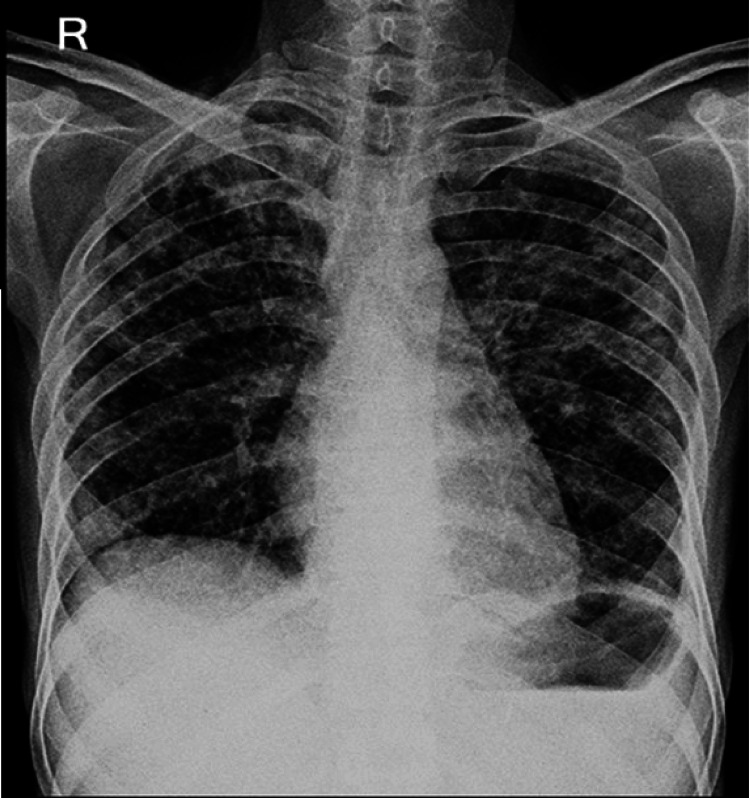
Chest X-ray (posteroanterior view) showing bilateral multiple reticulonodular infiltrates in the upper, mid, and lower zones.
